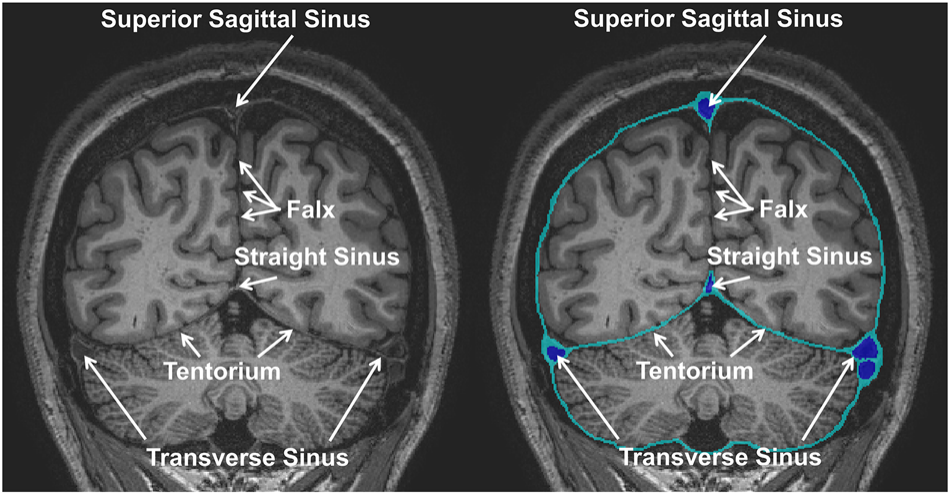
Segmentation of the dura mater. Coronal T1-weighted MRI (left) and outlines of the dura mater (right) with the principal dural reflections and sinuses. The falx cerebri, which separates the two cerebral hemispheres, was only partially visible on the images and was not segmented. The tentorium cerebelli was manually segmented. The superior sagittal sinus, transverse sinuses, and straight sinus—found along the attached edge of the falx, the line of attachment of the tentorium, and the line of attachment of the falx to the tentorium, respectively—were modeled as 500 μm thick outer layers enveloping the large venous vessels visible in the MRA dataset.
